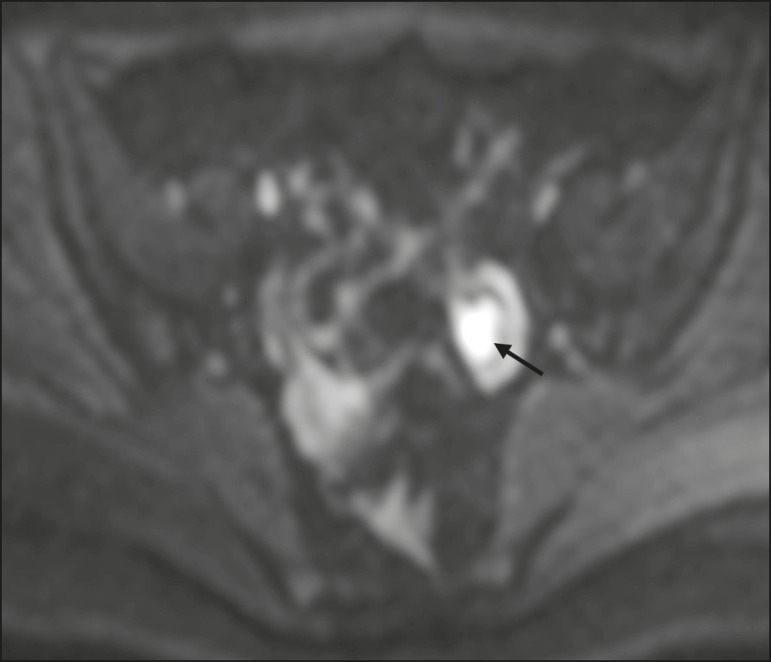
MRI of a female patient with a hemorrhagic cyst. Axial DWI at b = 1000
